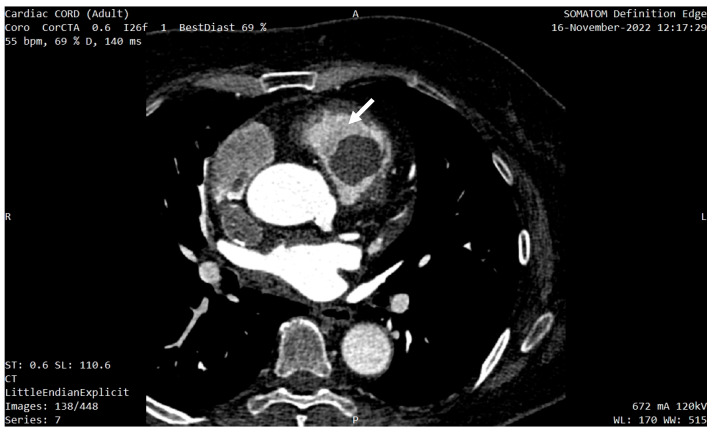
Cardiac CT showing the mass localization 3 mm anterior of the pulmonary valve (white arrow), occupying 80% of the RVOT.
